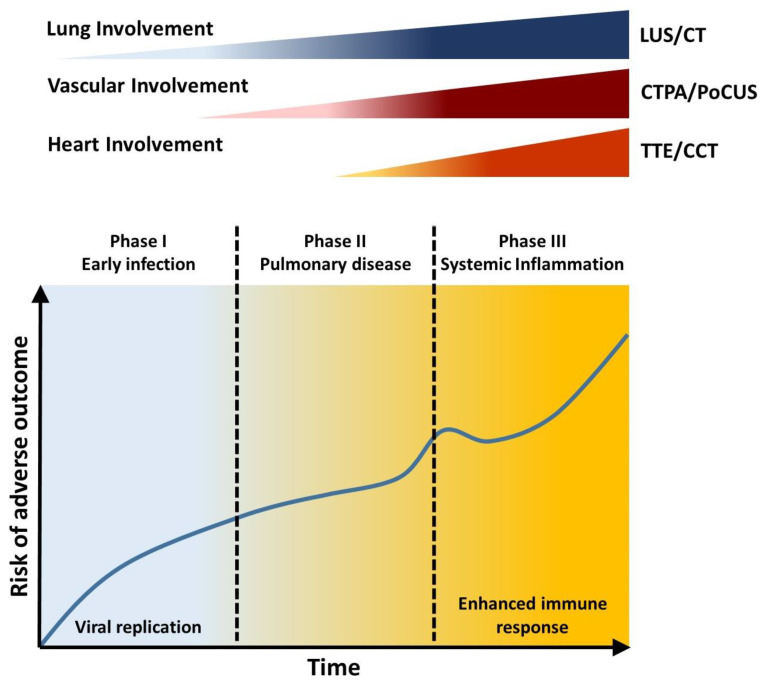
Viral replication and host immune response cooperate in disease progression. Through the three stages, different chest imaging modalities are useful to study the cardiovascular involvement. Transthoracic echocardiography (TTE) can identify left ventricle impairment as well as increased pulmonary hypertension and right ventricular dysfunction. Viral infection or to systemic inflammation can induce cardiovascular complications at different stages of the disease, inducing an increased risk for adverse outcome. To assess cardiovascular involvement specific multimodality imaging is required. CT: computed tomography; CTPA: computed tomography pulmonary arteriography; LUS: lung ultrasound; TTE: transthoracic echocardiography.
